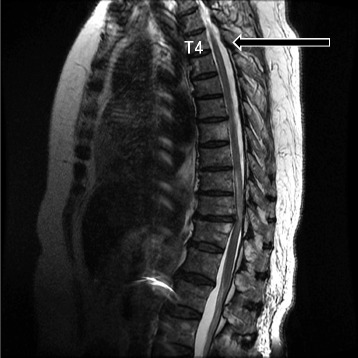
One week after the operation, sagittal MRI revealed that the hyperintense region had extended to approximately the T4 level
